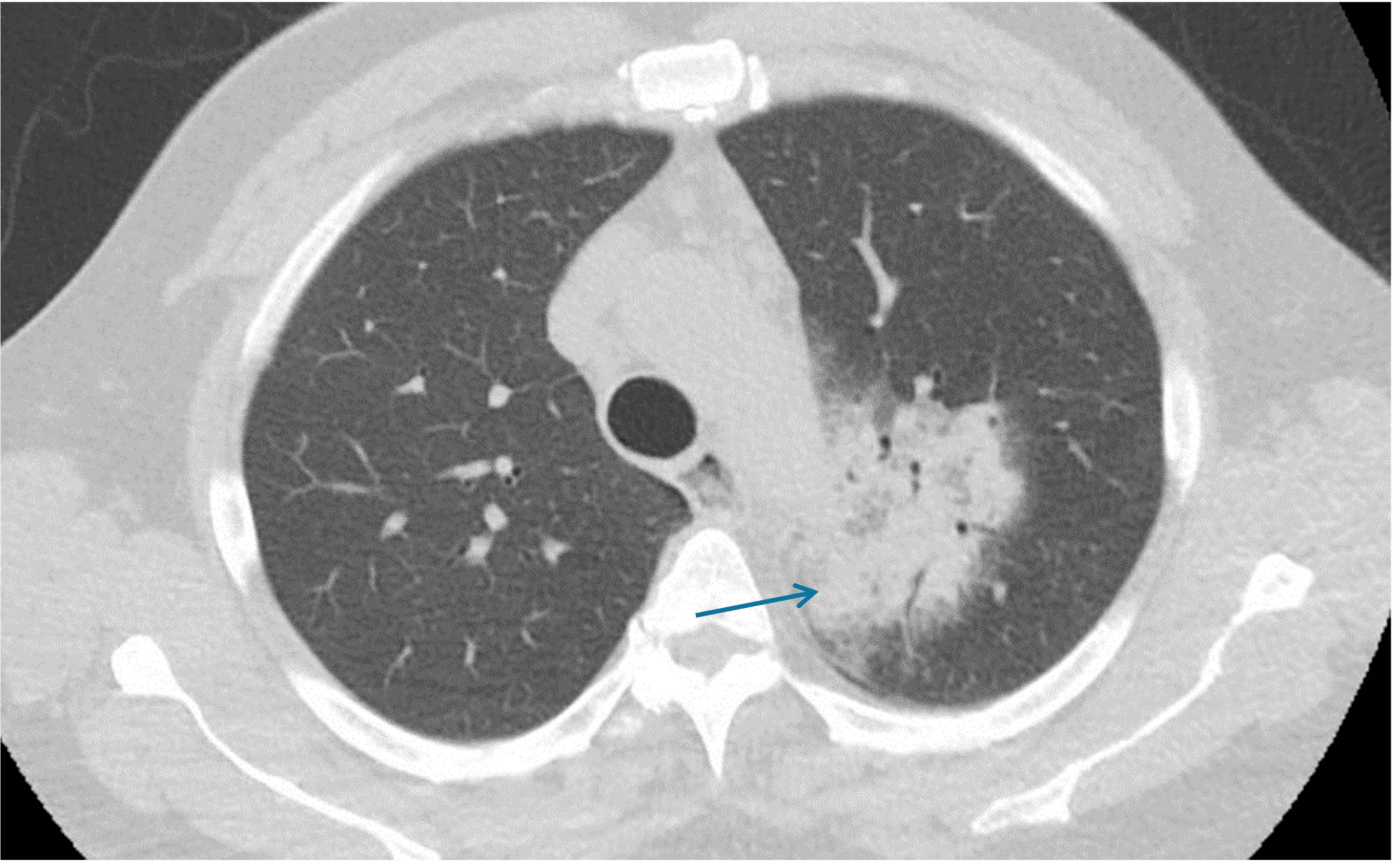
CT scan image at the time of presentation Mass-like opacity within the left upper lobe (LUL), 7.2 x 5.5 x 7.8 cm in size, along with mediastinal lymphadenopathy.
